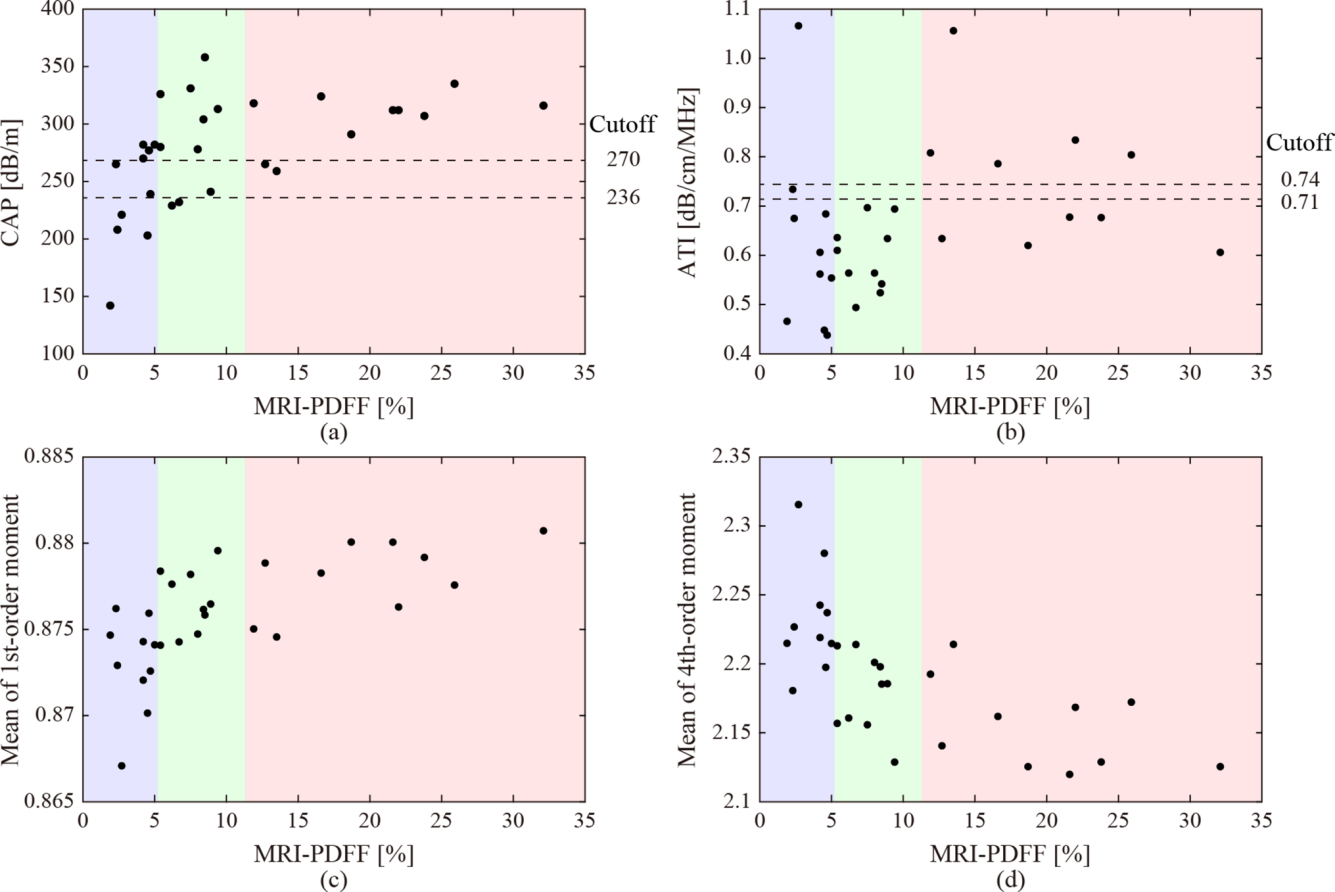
Relationship between hepatic fat deposition (MRI-PDFF) with ultrasound attenuation of a CAP, b ATI, and ES of the means of c first- and d fourth-order moments in all the ultrasound images
